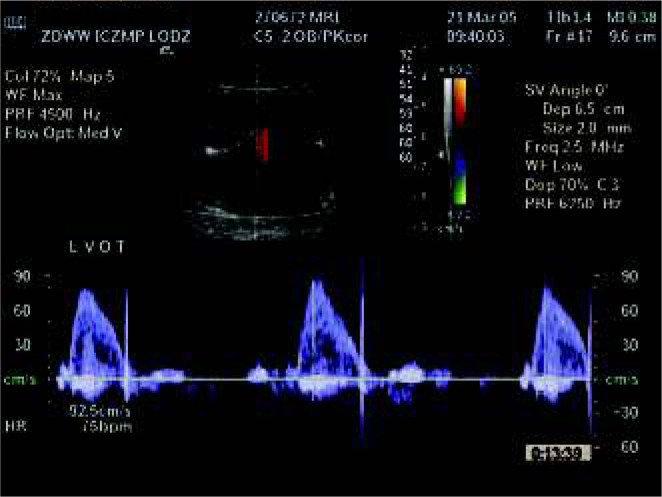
Isolated complete heart block in Doppler evaluation in a fetus with normal heart anatomy. Ryc. 6. Izolowany blok serca płodu (przy prawidłowej budowie serca) w badaniu za pomocą Dopplera.
